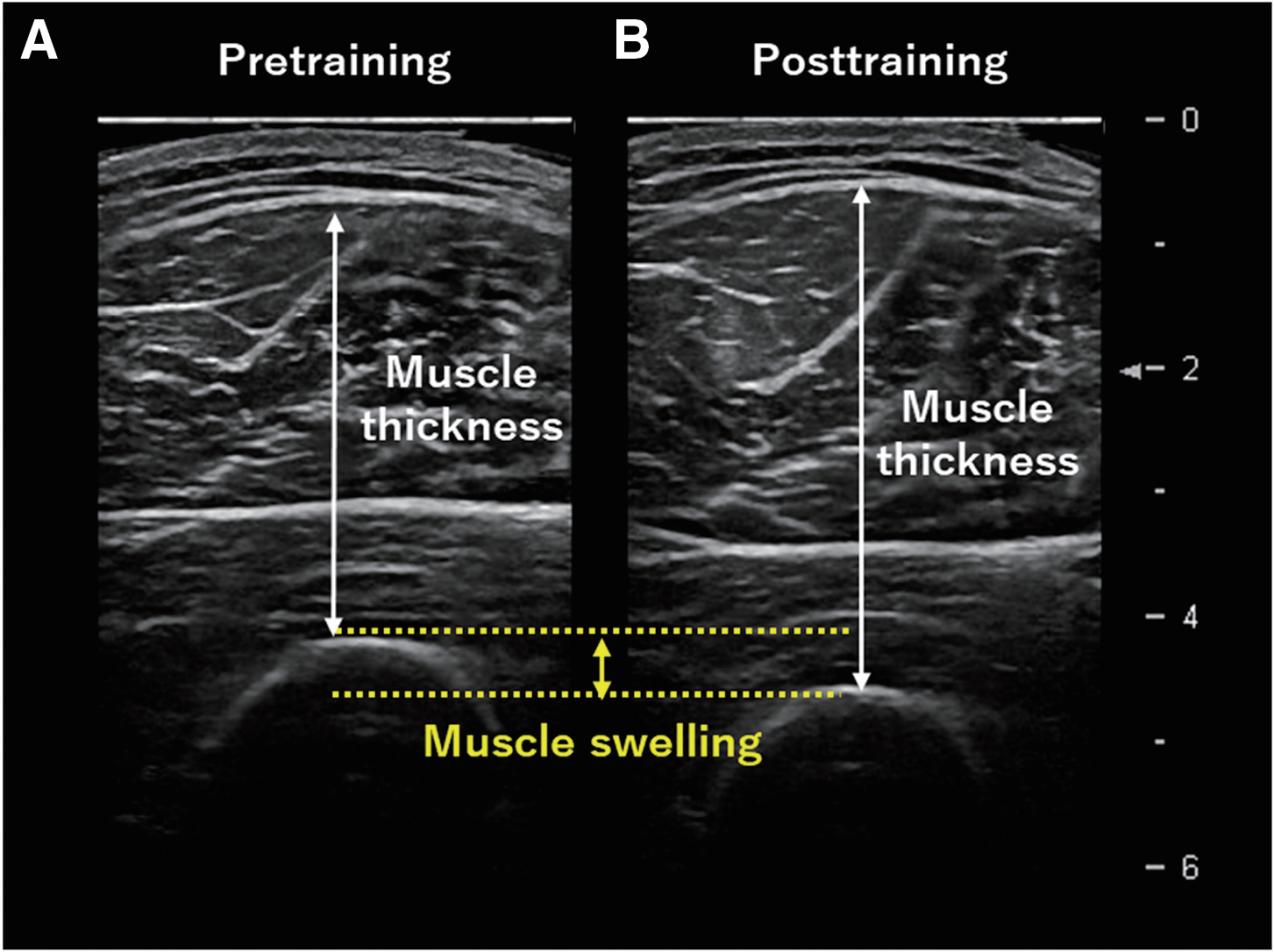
Immediate changes in MT and EI with resistance training An increase in MT can be confirmed in post-resistance training (B) compared to pre-resistance training (A) using B-mode ultrasonography. Muscle swelling, defined as the augmentation in MT following resistance training, is observed concurrently with an increase in EI, This immediate higher EI is associated with delayed onset muscle soreness. MT, muscle thickness; EI, echo intensity
